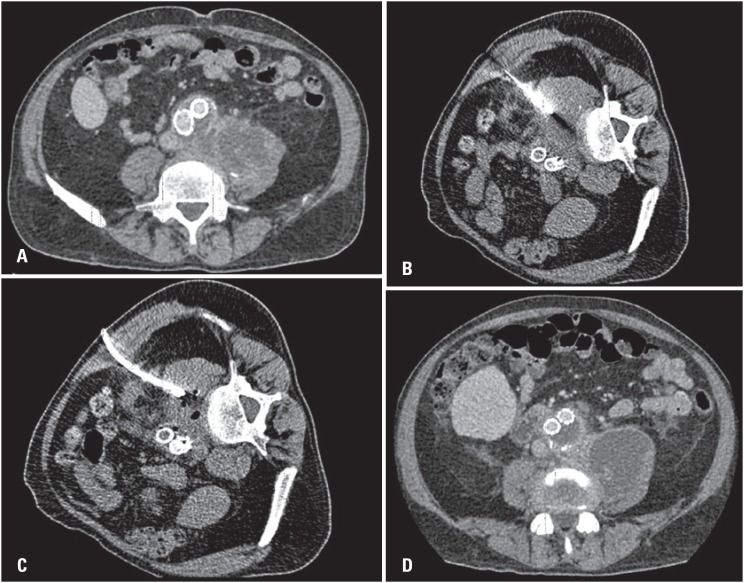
Contrast-enhanced abdominal computed tomography. (A) A fluid collection inside left psoas, surrounding aortoiliac graft, (B) Percutaneous computed tomography-guided drainage was performed using Seldinger technique, (C) Final imaging control demonstrating a well-positioned Dawson-Mueller drain, (D) Detection of a new fluid collection on the same site
